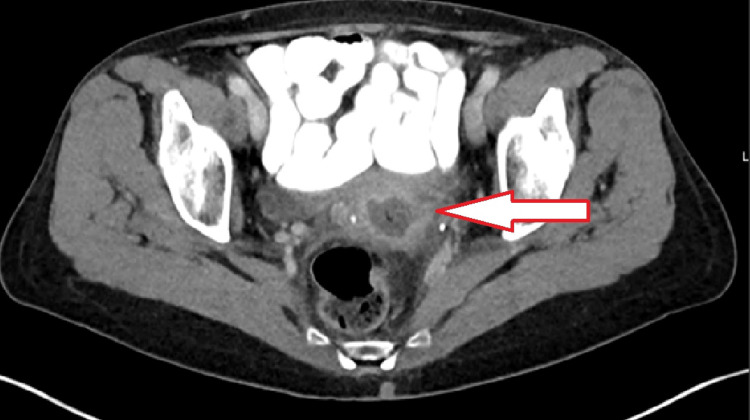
Axial cut of CT pelvis showing a fluid collection in cervix stroma
